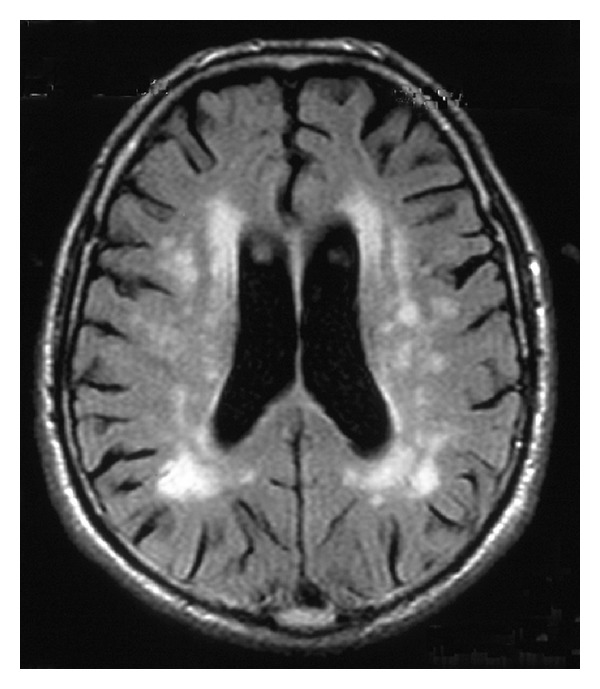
Axial FLAIR MRI of the brain showing periventricular hyperintense areas in a patient with chronic HCV infection and cognitive changes.
